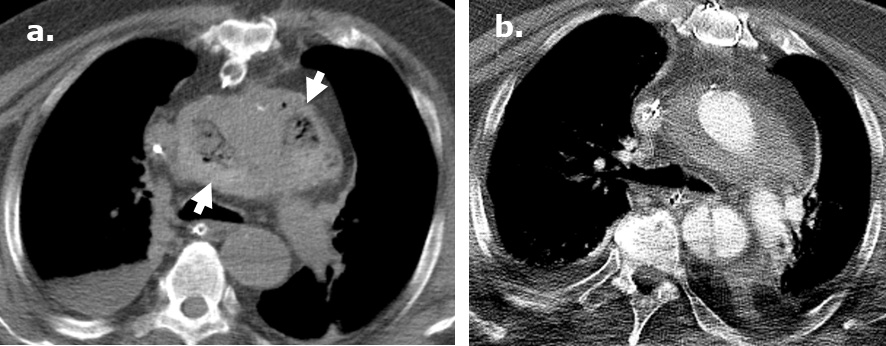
Longitudinal postoperative changes of Surgicel following type A aortic dissection repair. An axial image from an unenhanced CT (a) performed in a 71-year-old male at post-operative day ^®^ following a type A dissection repair demonstrates a heterogeneous gas-containing collection surrounding the aortic root in keeping with Surgicel. Subsequent axial intravenous-enhanced CT (b) performed at post-operative day 11 reveals near resolution of the Surgicel with evolution to a homogenous perigraft collection typically seen in the post-operative setting.
